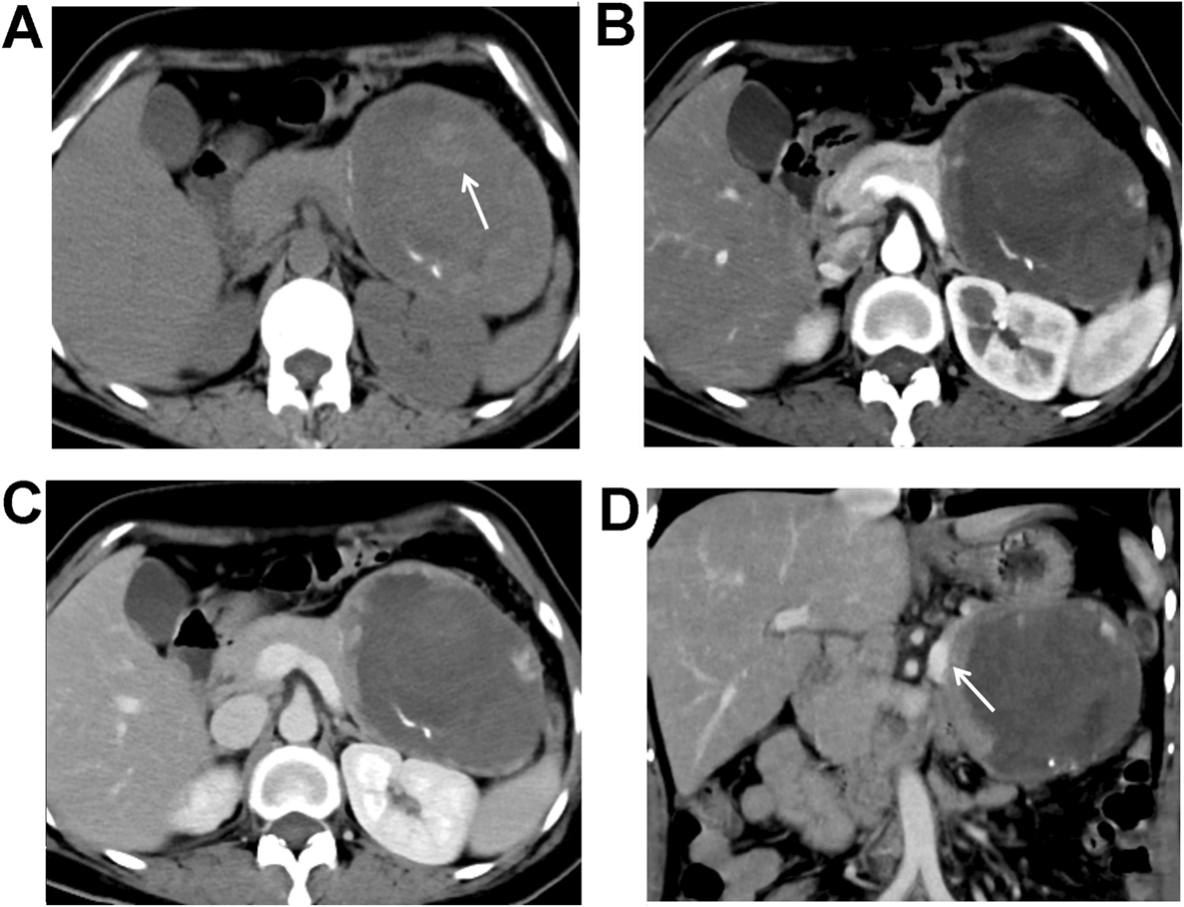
A 50-year-old female patient with pain in the upper abdomen. Plain CT scanning + enhanced scanning showed pseudo-papillary neoplasm of pancreas (SPNP). a Plain scanning showed a huge oval cystic and solid mass at the pancreatic tail, of which the boundaries were clear. Punctate calcifications were found around the mass. Small patchy bleeding focuses were found in the mass (white arrow). b Low enhancement of the lesion was found in the arterial phase, which was lower than the normal pancreas, and showed “ball-holding” changes with the pancreatic body. c Persistent enhancement of the solid components was found in the portal venous phase, which showed “floating cloud” sign. d Reconstructed coronal image showed compressing and circuity of the splenic vein (white arrow)
